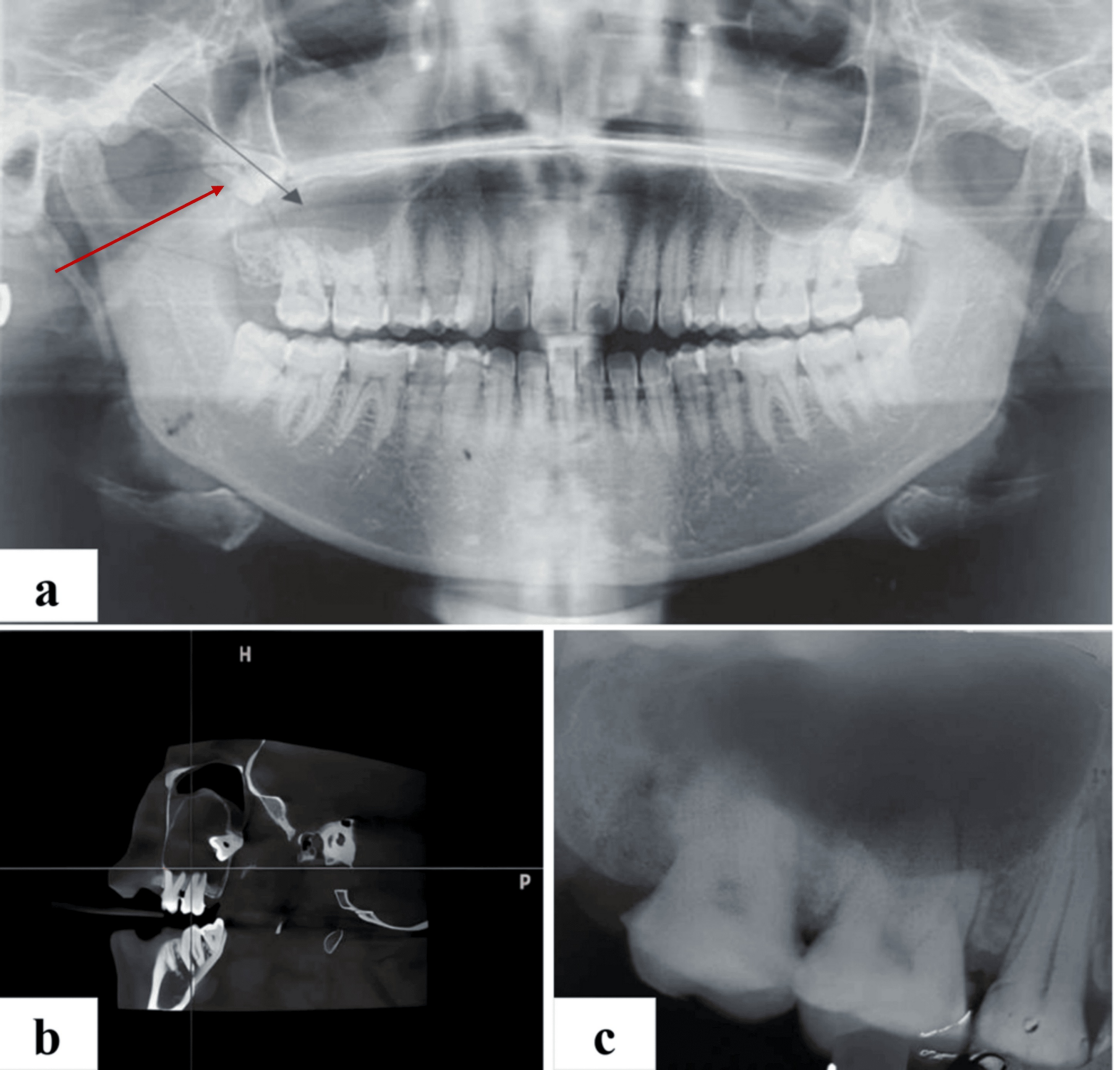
(a) Preoperative orthopantomograph (OPG) showing unilocular well defined radiolucency in maxillary posterior tooth region. (b) CBCT showing unilocular lesion in right posterior maxilla. (c) Intraoral periapical radiograph showing well-defined radiolucency. CVCT: cone-beam computed tomography
